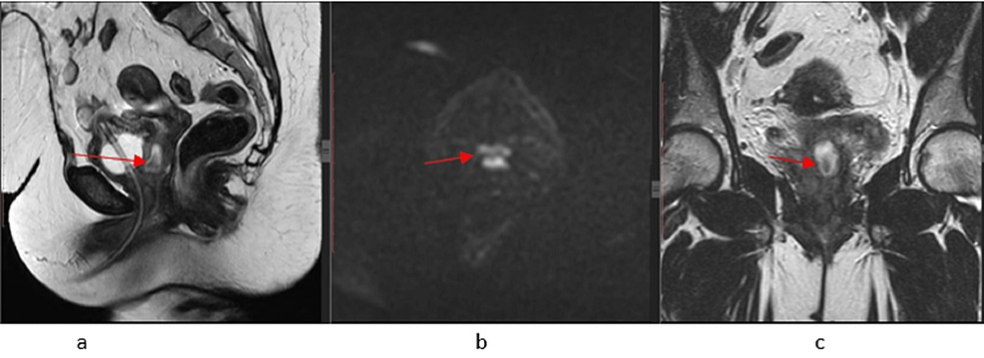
On subsequent MRI while ongoing chemotherapy demonstrates partial treatment response with a significant interval decrease in the size of the soft tissue hyperintense mass involving the upper two-thirds of the vagina as shown in T2 sagittal and coronal images (3a and 3c), which is showing restricted diffusion on DWI (3b); no enlarged pelvic side wall nodes were seen DWI: diffusion-weighted imaging
